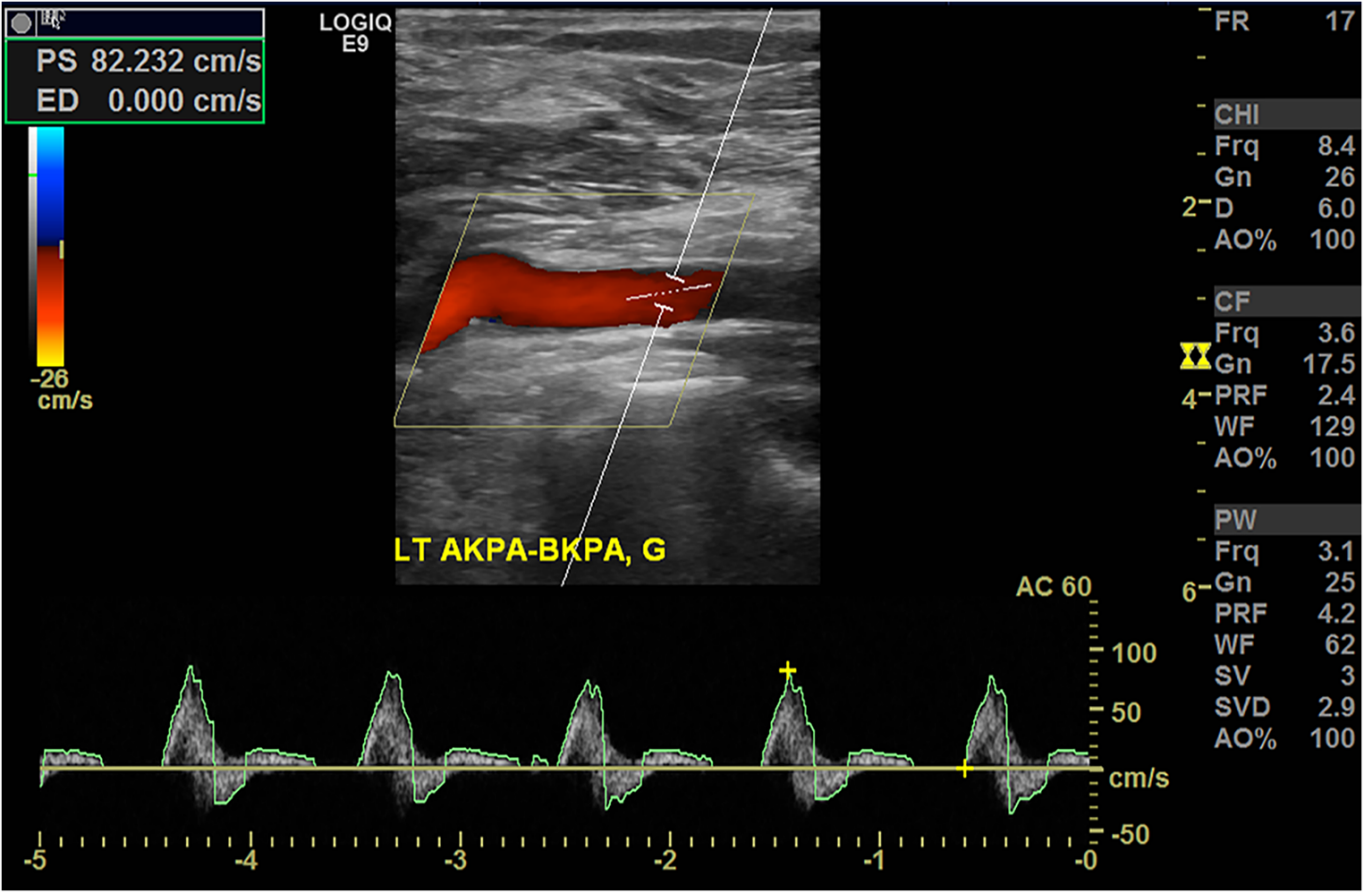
Duplex ultrasonography conducted 5 years after the operation demonstrating a patent interposition graft without stenosis.
